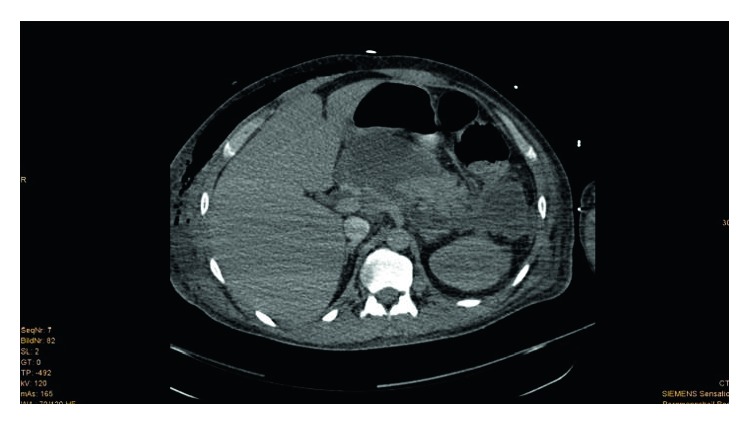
(Patient 1, scan 1) 18 days after trauma. CT scan of the abdomen showing pancreatitis with large expanding pseudocysts with a maximum diameter of 8 cm in the pancreas head-corpus transition area. Pigtail drainage was placed with guidance from CT in the fluid accumulation/pseudocyst from the left flank side. The drainage system emptied brown-tinged serous fluid.
